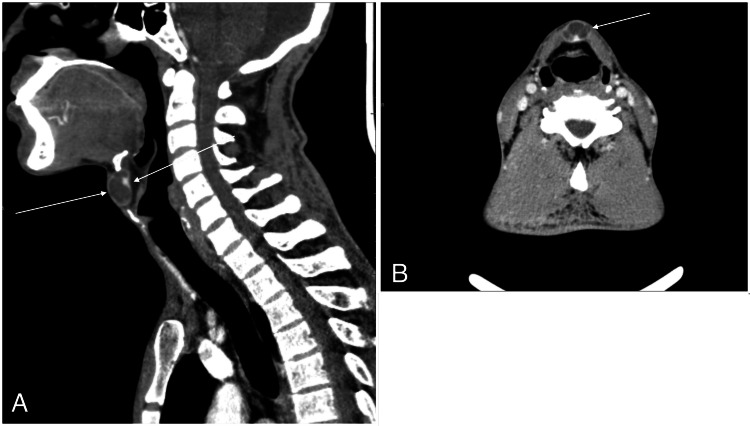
(A) Sagittal post contrast CT scan shows a complex cystic lesion situated in the upper anterior neck, in the midline, between the hyoid bone and the thyroid cartilage. It shows a thin enhancing wall, and a small enhancing nodule situated posteriorly. (B) Axial post contrast CT scan shows a complex cystic lesion situated in the anterior mid upper neck, with a thin enhancing wall and a small enhancing nodule situated posteriorly. CT: Computed tomography
